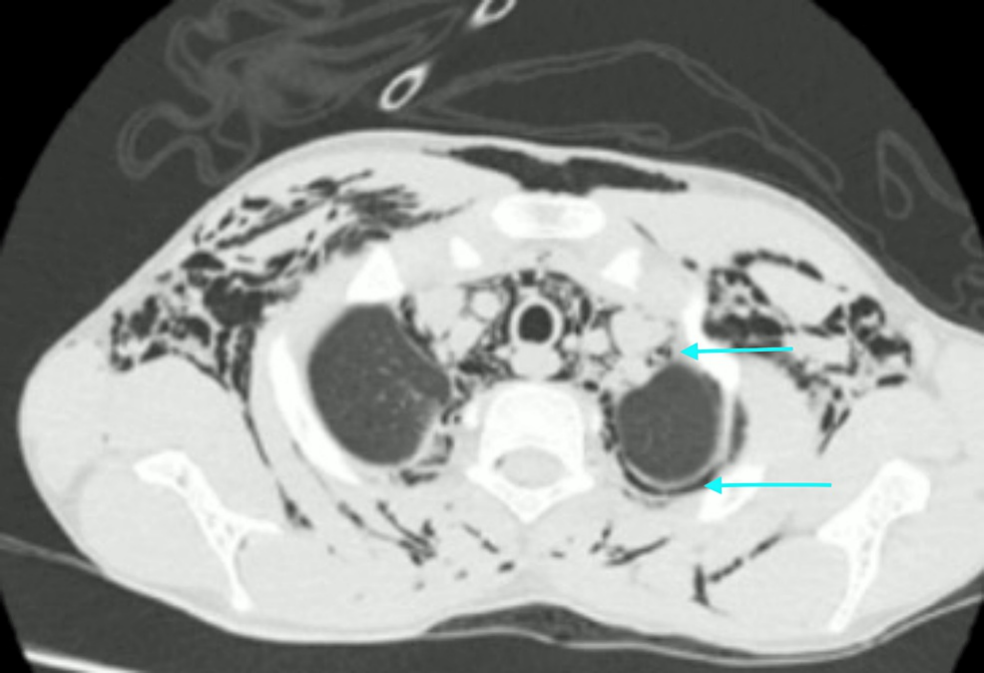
Axial sections through upper chest of HRCT There are tiny apical bullae (upper arrow) and pneumothorax on posterior aspect of left upper chest (lower arrow)
